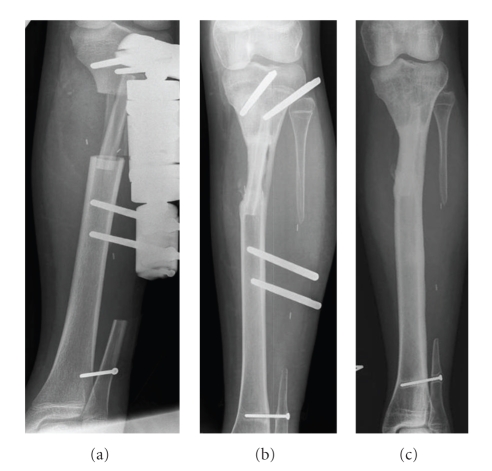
Resection of the proximal part of the tibial diaphysis performed because of an osteosarcoma and reconstruction of the bone defect with a double-barrelled pedicle vascularized fibula graft (patient 7). X-rays taken 9 days postoperatively (a), after 16.5 months when weight bearing without external fixation (fixation pins left until the effect of weight bearing was evaluated) had been started 3 weeks earlier (b), and status 53 months postoperatively (c).
